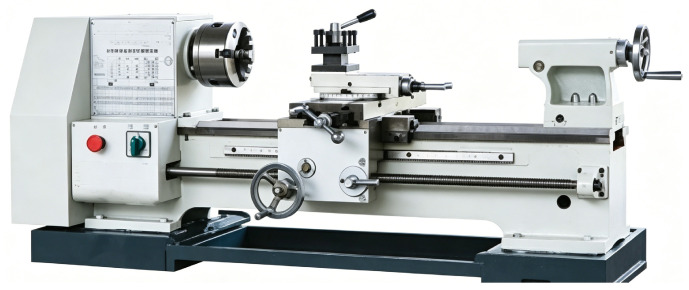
Roll ultrasonic testing experimental platform modified from a universal lathe.
